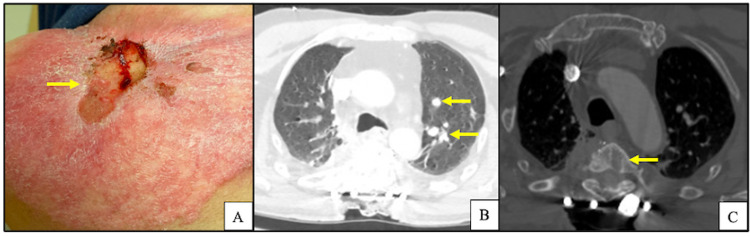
Gross pathology of BCC on the left shoulder at four-year follow-up; B) chest CT scan in lung window demonstrating stable to mildly progressed pulmonary nodules 48 months after initial diagnosis; C) chest CT scan in bone window demonstrating lytic changes at T2/T3 level 48 months after initial diagnosis. Yellow arrows indicate abnormal pathology.
